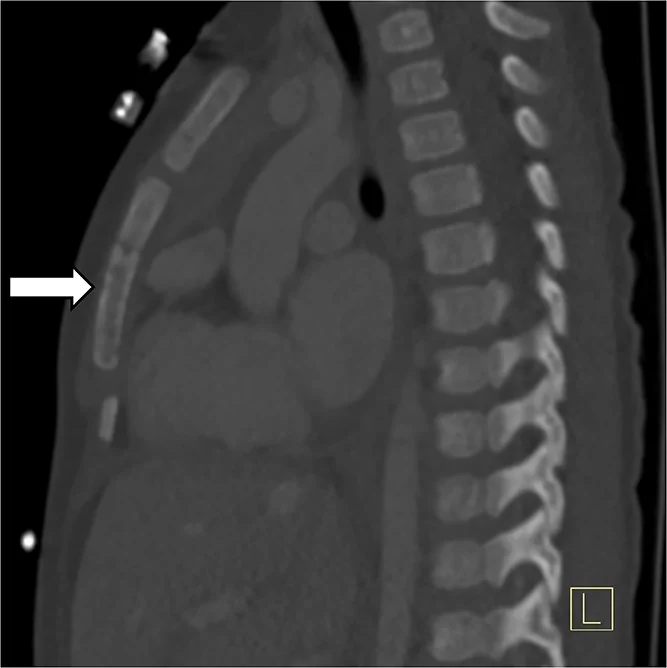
Sagittal CT image of the chest demonstrates bone marrow metastasis in the body of the sternum.
